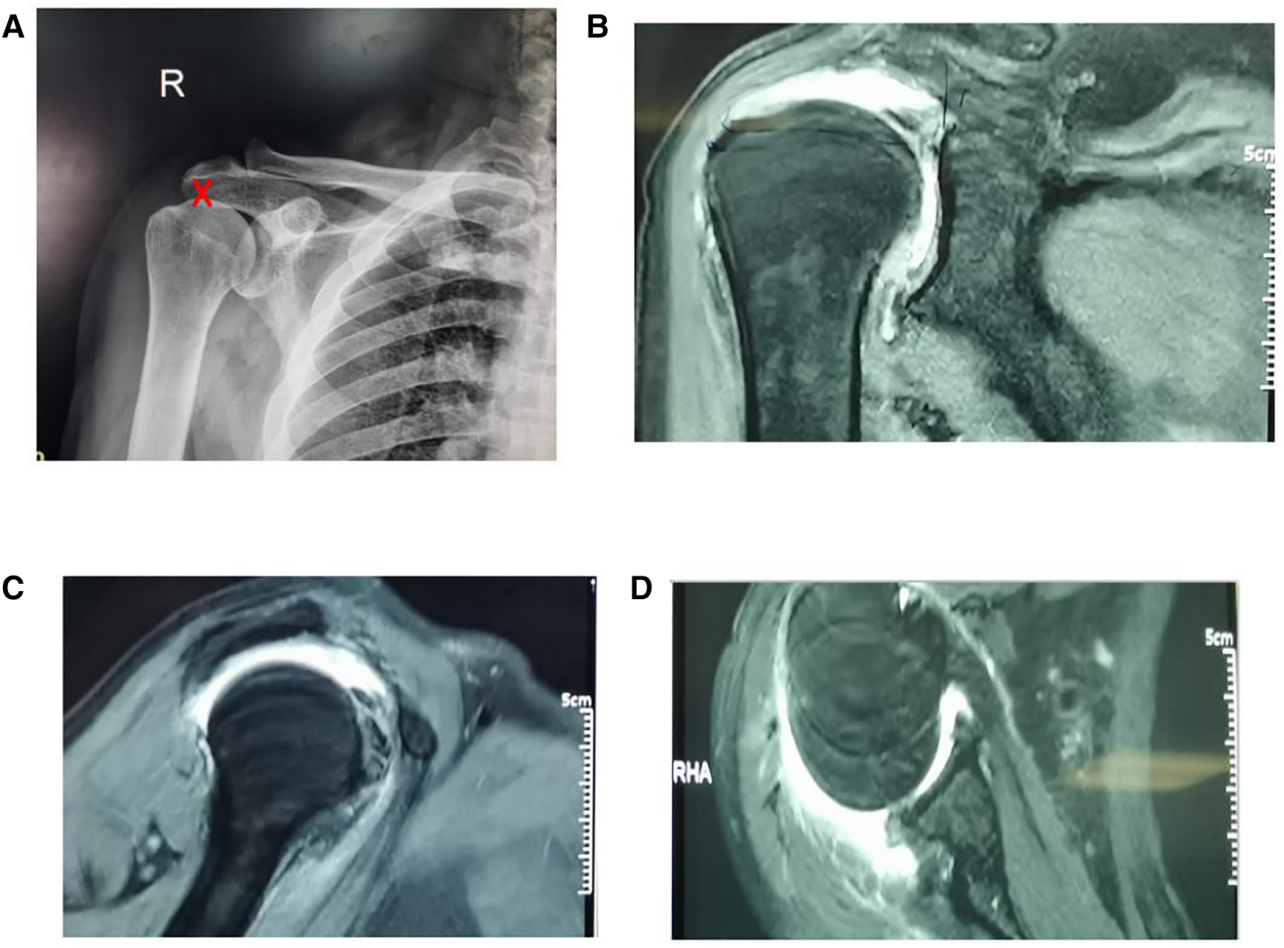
Preoperative radiograph of irreparable rotator cuff tears. (A) Red mark on the X-ray shows that the acromiohumeral distance (AHD) is 3.5 mm; (B–D) show huge irreparable rotator cuff tears.
